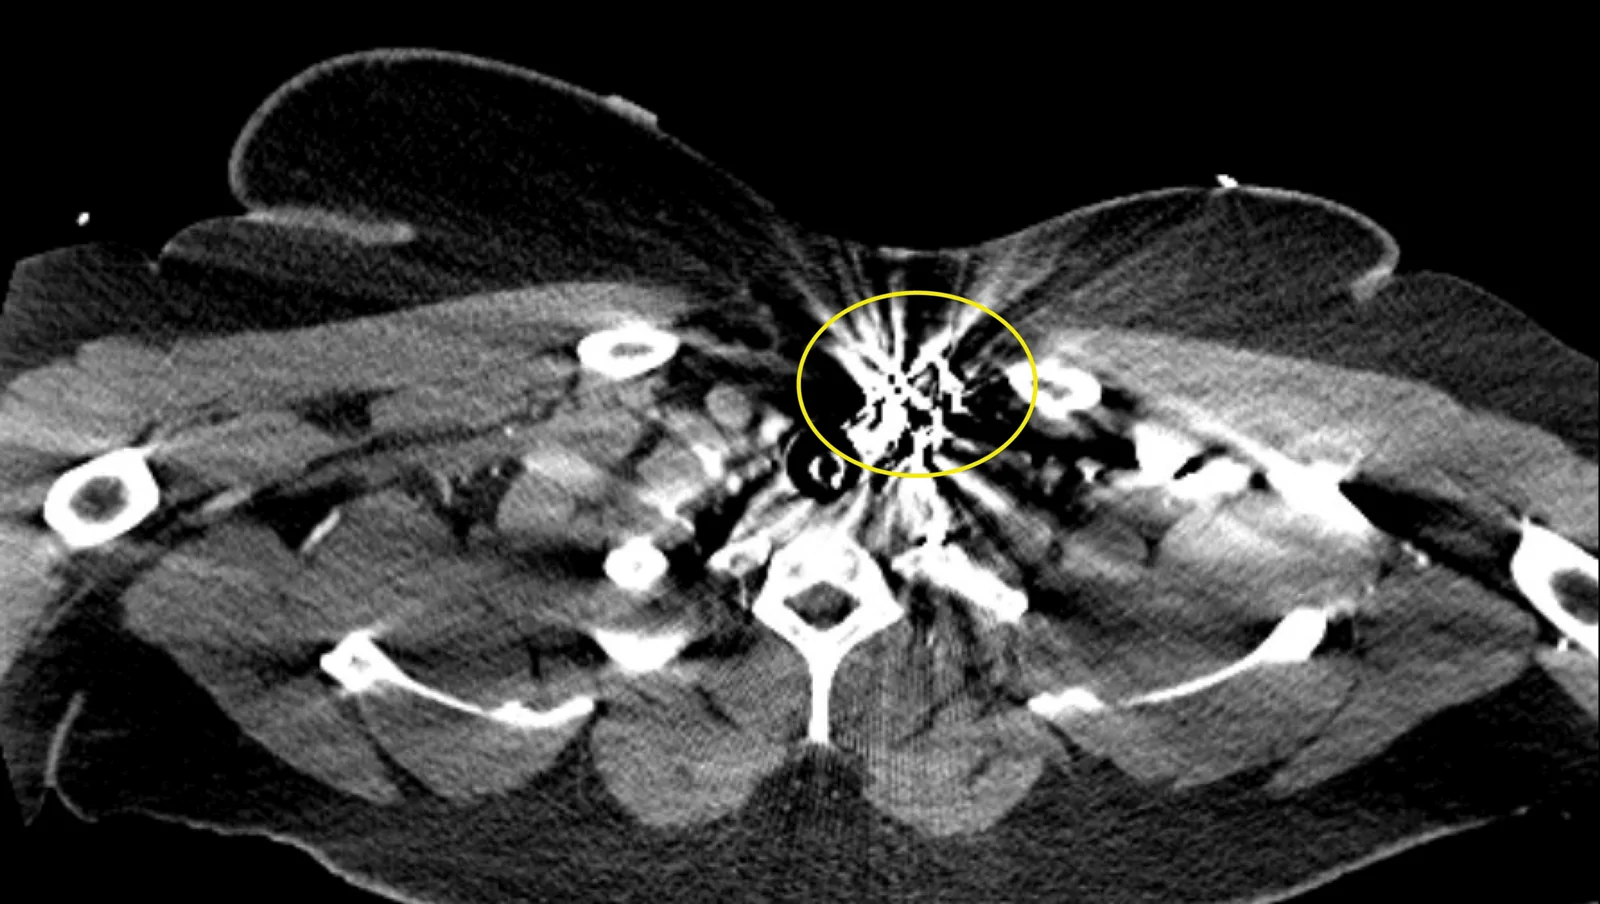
Representative CT image showing birdshot causing artifact over the carotid artery and jugular vein, obscuring visualization of vessel integrity.
